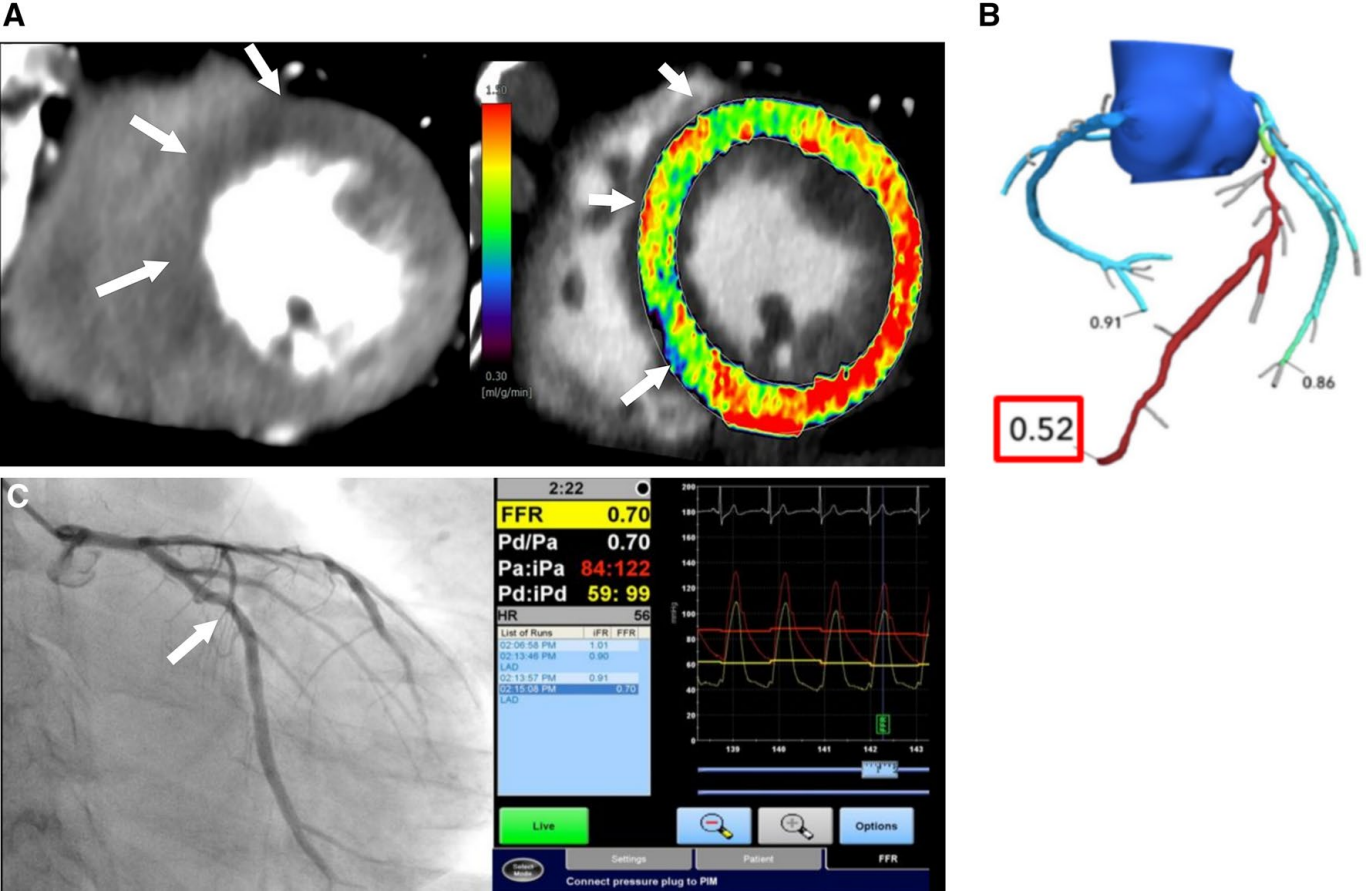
CTP versus CT-FFR. a CTP image and CT-MBF map showing a perfusion defect and lower CT-MBF in the anteroseptal wall (arrows). b A CT-FFR image in the same patient showing abnormal CT-FFR with a low value of 0.52 at the LAD. c ICA showing a stenotic lesion in the mid LAD with an abnormal FFR of 0.70. *CTP* computed tomography perfusion, *CT-MBF* computed tomography derived-myocardial blood flow, *CT* computed tomography, *FFR* fractional flow reserve, *ICA* invasive coronary angiography, *LAD* left anterior descending artery
